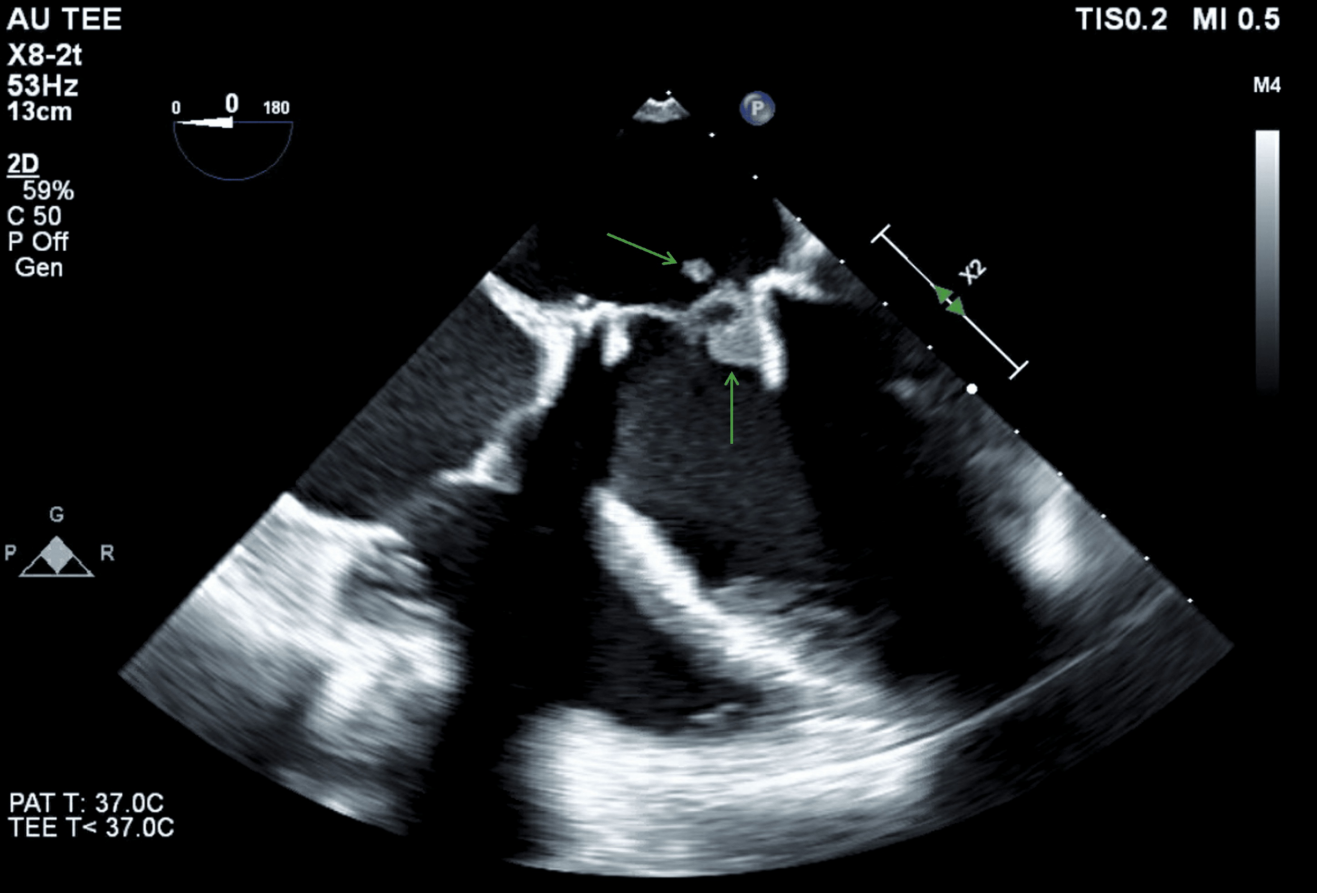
Transesophageal echocardiogram demonstrating a mobile hypodensity on the posterior leaflet and strut of the bioprosthetic Medtronic 29 mm mitral valve
